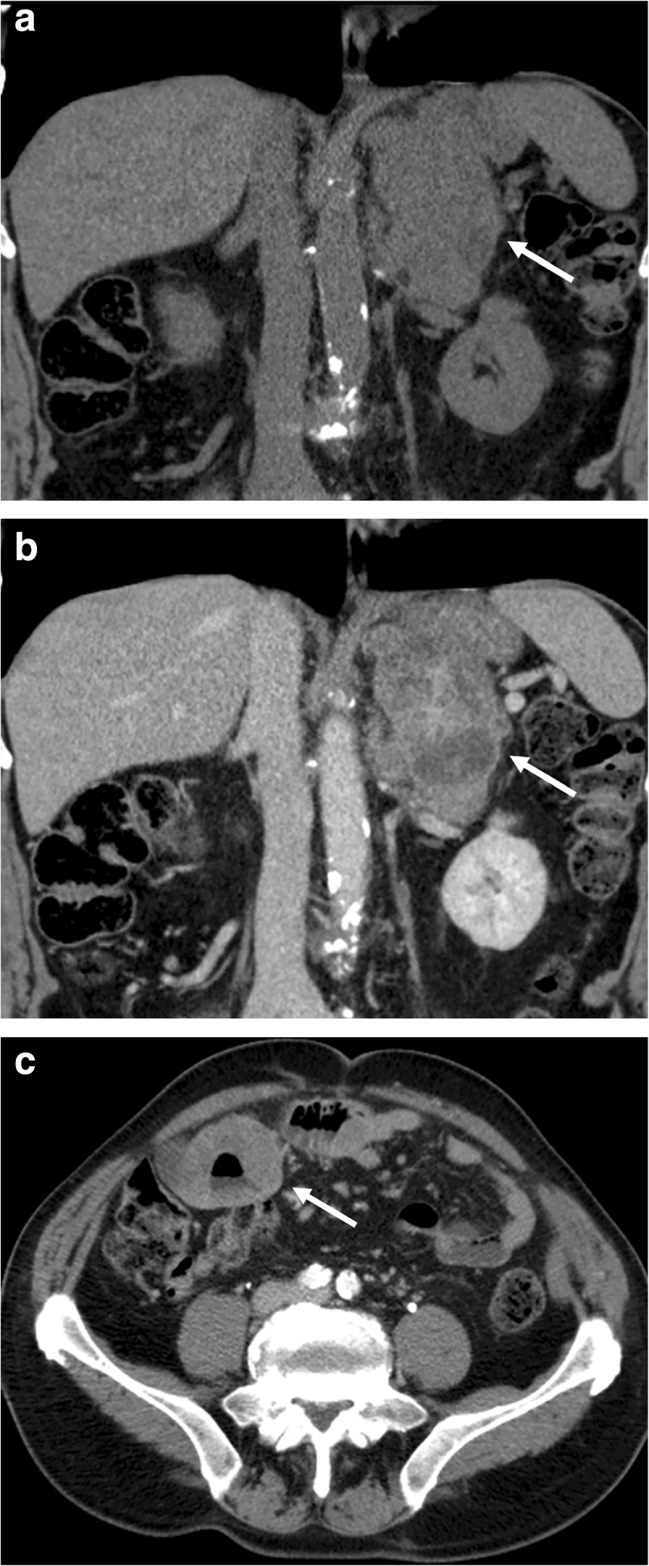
Left adrenal lymphomatous lesion in a 70-year-old man with non-Hodgkin lymphoma. Coronal reformatted CT images before (a) and after contrast injection in portal phase (b) show a large left adrenal mass (arrow) with inhomogeneous enhancement. Axial CT image of the lower abdomen in portal phase (c; arrow) shows the gastrointestinal involvement by lymphoma as aneurysmal dilatation of some loops of the small bowel
